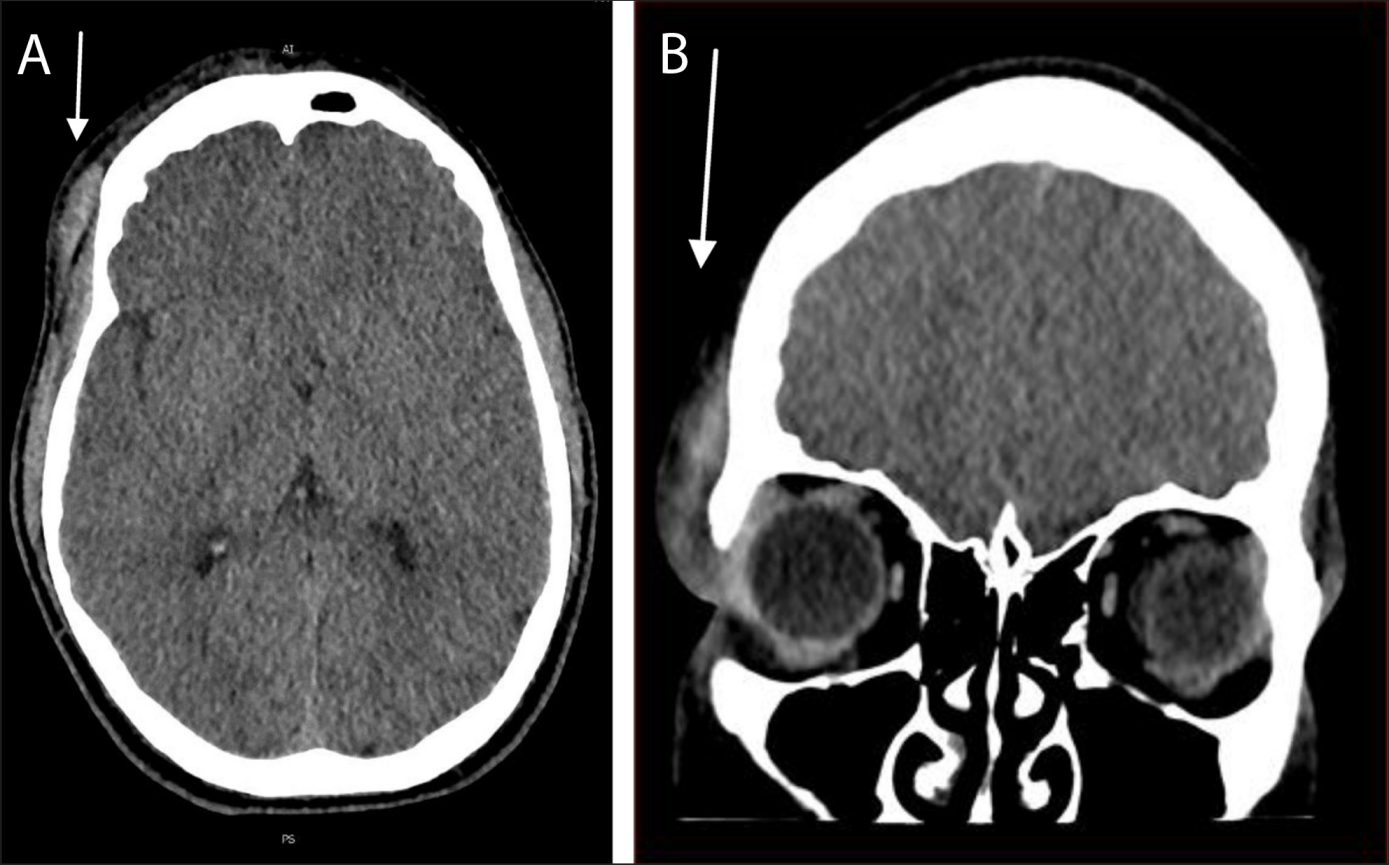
Seven weeks post trauma, the boy presented with a slow growing, pulsatile, compressible, painless mass in the right temporal area. B-mode ultrasound revealed a 1.1 × 0.9 × 0.5 cm saccular central hypoechoic structure with peripheral hyperechogenicity (Figure 2A). Color-Doppler ultrasound (CDUS) revealed a vascular structure associated with and displacing the superficial temporal artery (Figure 2B) with an embedded yin-yang sign suggestive of ‘to-and-fro flow’ (Figure 2C). The image was compatible with a partially thrombosed pseudoaneurysm of the superficial temporal artery.
